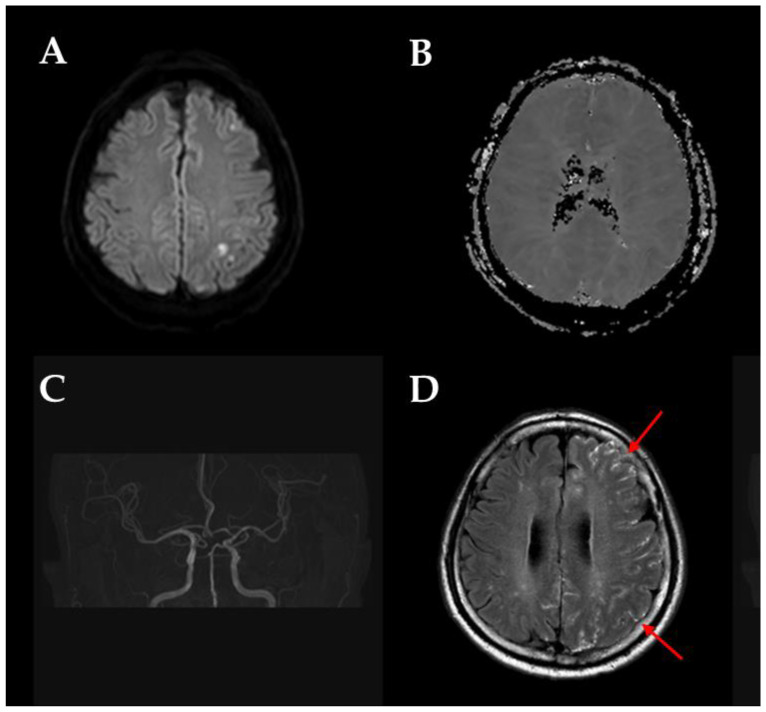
A patient presenting with acute-onset right-sided weakness. (A,B) DWI and apparent diffusion coefficient (ADC) images show an acute infarction in the left parietal lobe. (C) Intracranial time-of-flight angiography reveals no evidence of stenosis or occlusion. (D) Post-contrast FLAIR image demonstrates diffuse sulcal enhancement (Hyperintense Acute Reperfusion Marker sign) in the left cerebral sulci (arrows).
